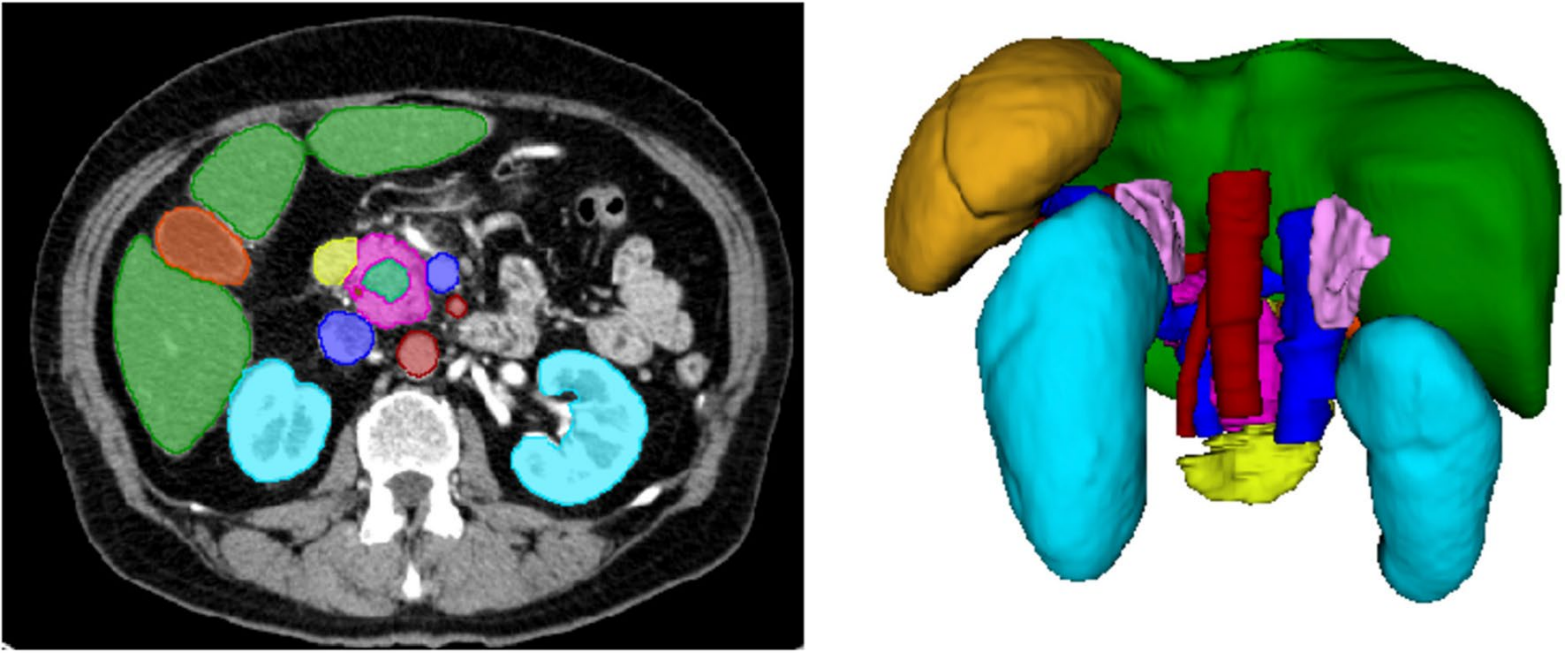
Example of a manual segmentation of anatomical structures within a late-arterial phase computed tomography scan. Turquoise = PDAC tumor; red = arteries (aorta, celiac trunk, hepatic artery, splenic artery, and superior mesenteric artery); dark blue = veins (inferior vena cava, portal vein, splenic vein, and superior mesenteric vein); pink = pancreas; yellow = duodenum; green = liver; orange = gallbladder. *PDAC*, Pancreatic ductal adenocarcinoma
